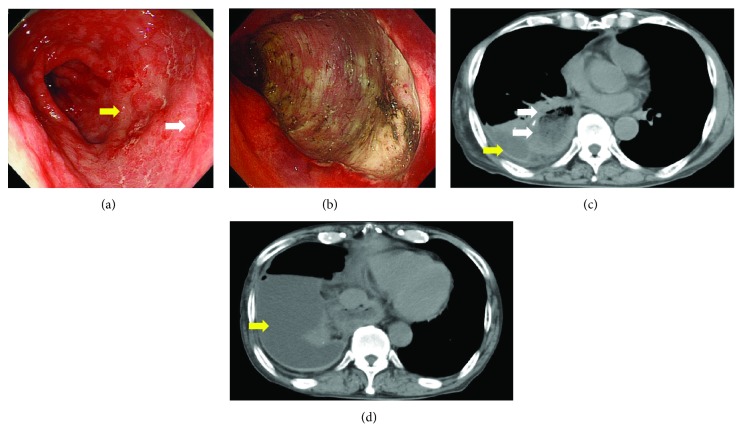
(a) An endoscopic image obtained during endoscopic submucosal dissection (ESD). White light imaging revealed 2 synchronous gastric tube cancers (yellow and white arrows) at the posterior wall of the lower gastric tube. (b) An endoscopic image obtained during ESD. Both lesions were resected *en bloc* in the same piece without perforation. (c) A computed tomography (CT) image obtained 2 days after ESD. CT revealed right pleural effusion (yellow arrow). The dilated and tortuous gastric tube in the posterior mediastinal reconstruction route markedly protruded into the right thoracic cavity, close to the pleura (white arrow). (d) A CT image obtained 3 weeks after ESD. CT revealed pyothorax of the right chest (yellow arrow).
